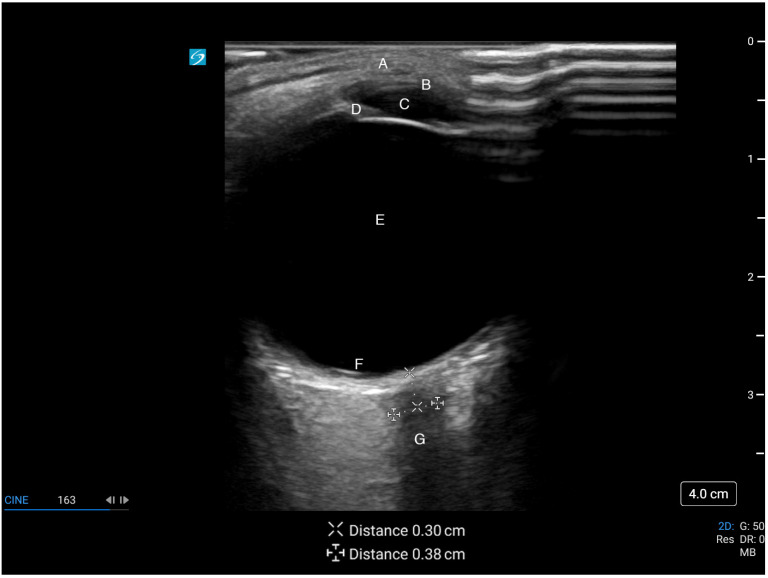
Ultrasound image of the eye and optic nerve. A: eyelid, B: cornea, C: anterior chamber, D: iris, E: vitreous chamber, F: retina, G: optic nerve with optic nerve sheath diameter of 3.8 mm, measured at 3 mm from the retina. This figure was obtained with consent from the model for publication.
